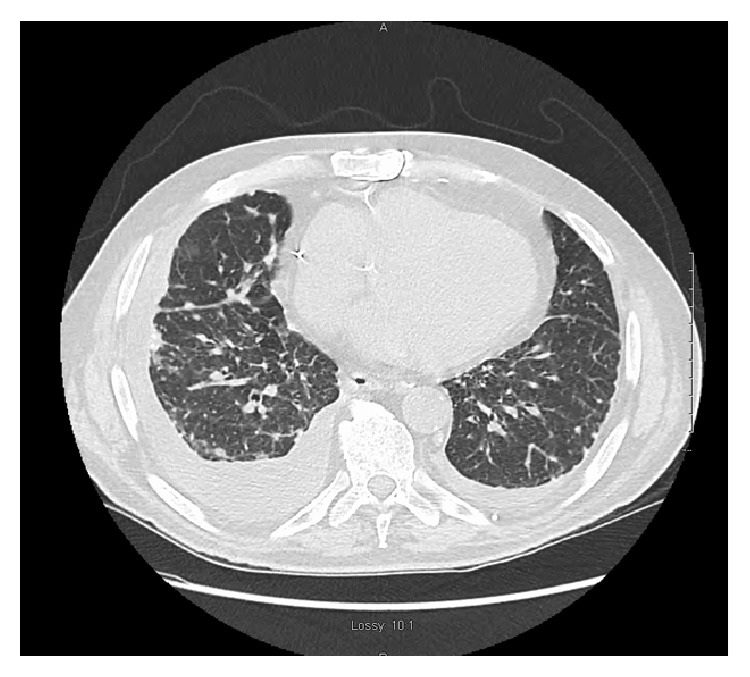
Chest CT showed moderate-sized bilateral pleural effusion and thickening with pulmonary nodules. There is mild interstitial fibrosis. There are multiple bilateral intrapulmonary nodules seen. Some of the nodules are pleural-based. A few nodules do have a small amount of associated calcification.
